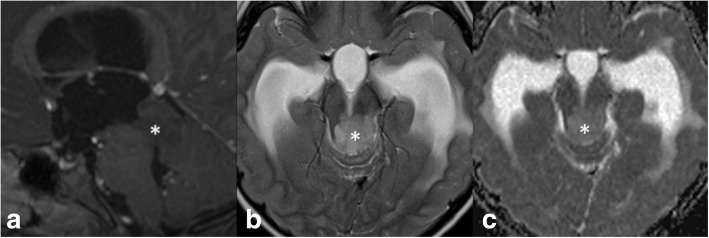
Typical MRI features of tectal glioma. a Sagittal post-contrast T1-weighted image shows a typical non-enhancing, T1 hypointense lesion obstructing the cerebral aqueduct (*). b Axial T2-weighted image shows typical T2 hyperintensity of the lesion (*), and periventricular CSF accumulation indicative of hydrocephalus. c on ADC map, tectal gliomas (*) are typically high in signal (“facilitated” diffusion)
